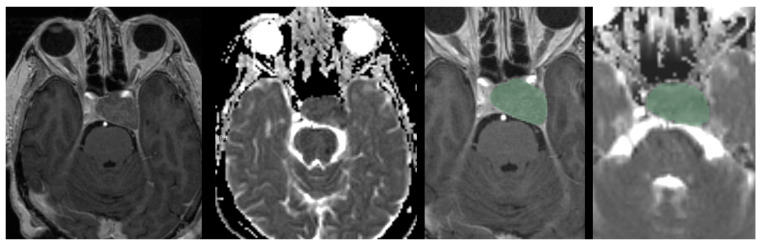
Representative segmentation example of a left cavernous sinus meningioma. Contrast-enhanced T1-weighted images and ADC maps are shown with the corresponding manually delineated whole-tumor segmentations.
